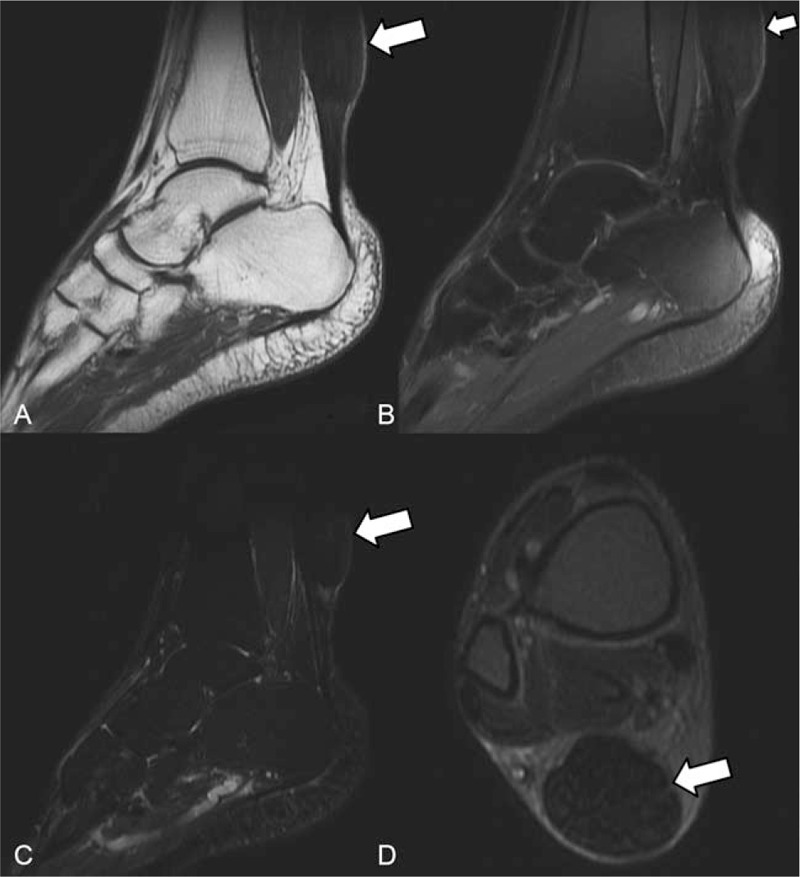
Achilles tendon xanthoma (right) in the patient. (A) Sagittal T1W, (B) proton density, and (C) STIR images show fusiform enlargement of the right Achilles tendon (arrows); the xanthoma is relatively isointense compared to muscle. (D) An axial proton density image shows diffuse fat infiltration with low-intensity tendon bundles interspersed within (arrows). STIR = short tau inversion recovery, T1W = T1-weighted.
